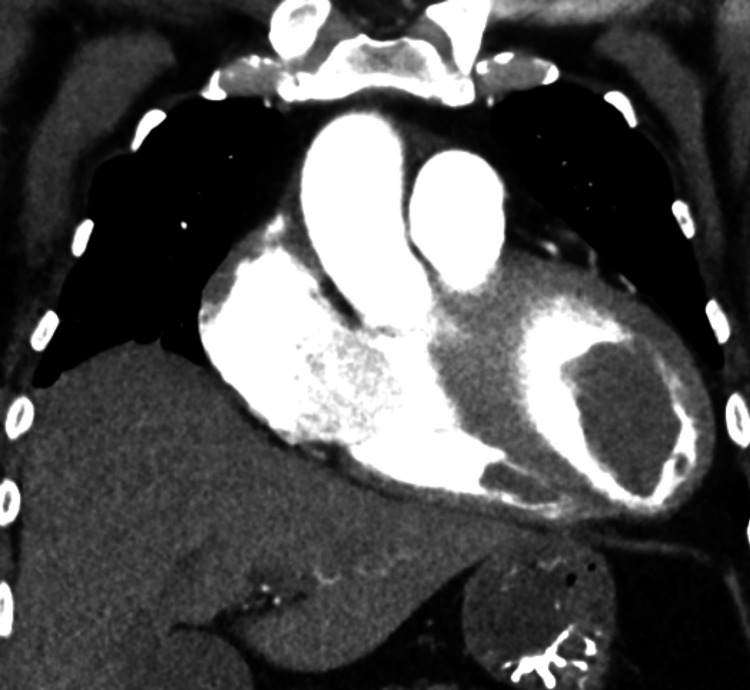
CT chest sagittal view of the left ventricular thrombus.
